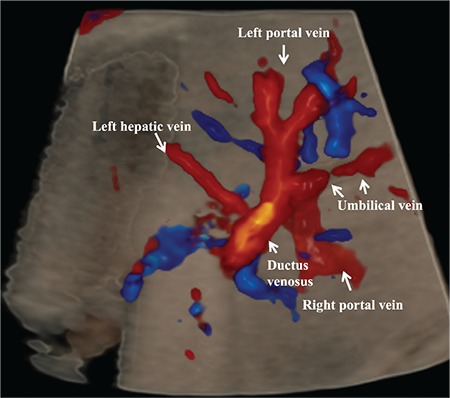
This figure shows a transverse view of the abdomen using three-dimensional imaging with a glass body and color Doppler. The aliasing signal identifies the actual location of the ductus venosus.
